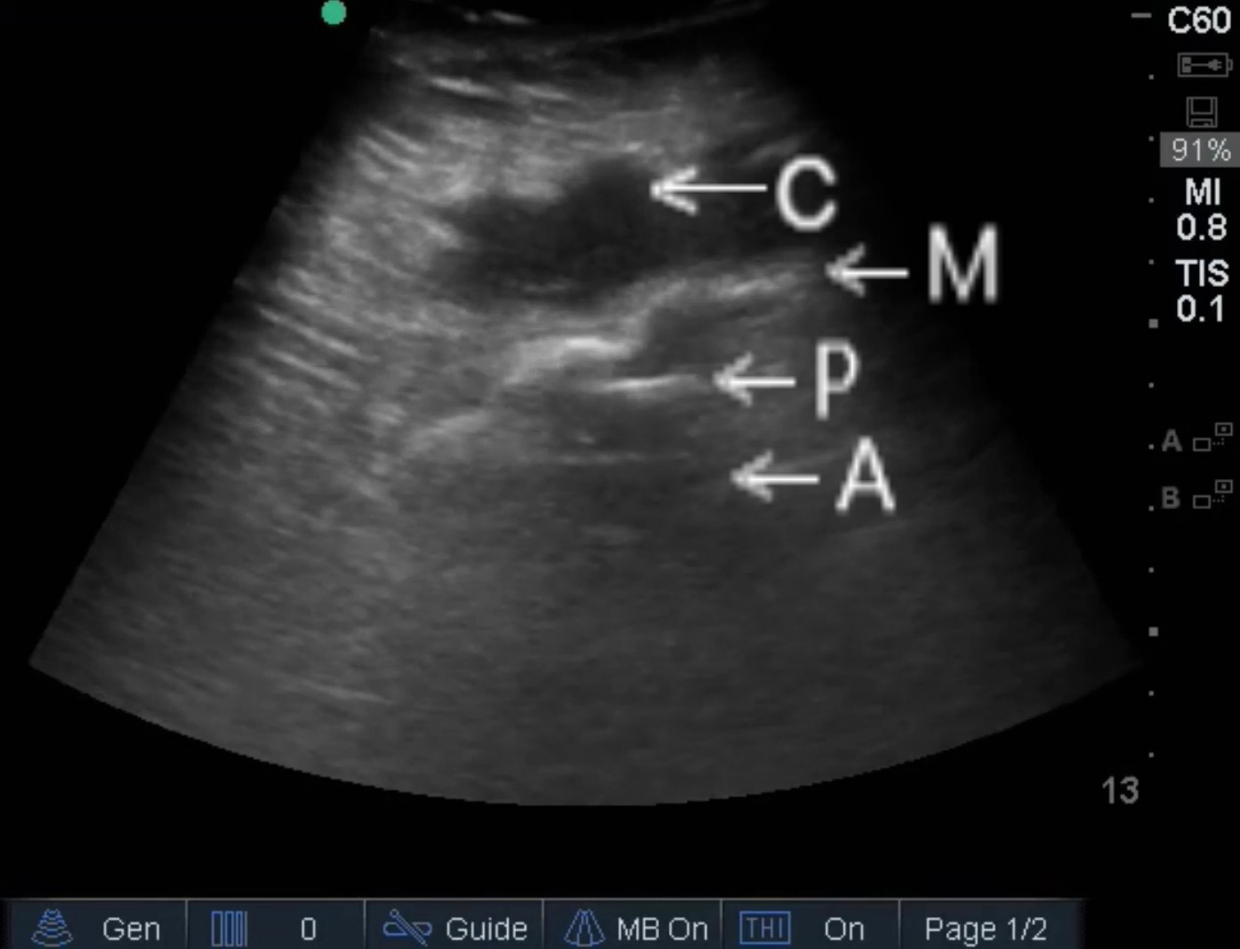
Point-of-care ultrasound of the abdominal wall abscess, demonstrating surgical mesh (M) and associated fluid collection (C) extending across the peritoneum (P) and communicating with intraperitoneal abscess (A).
